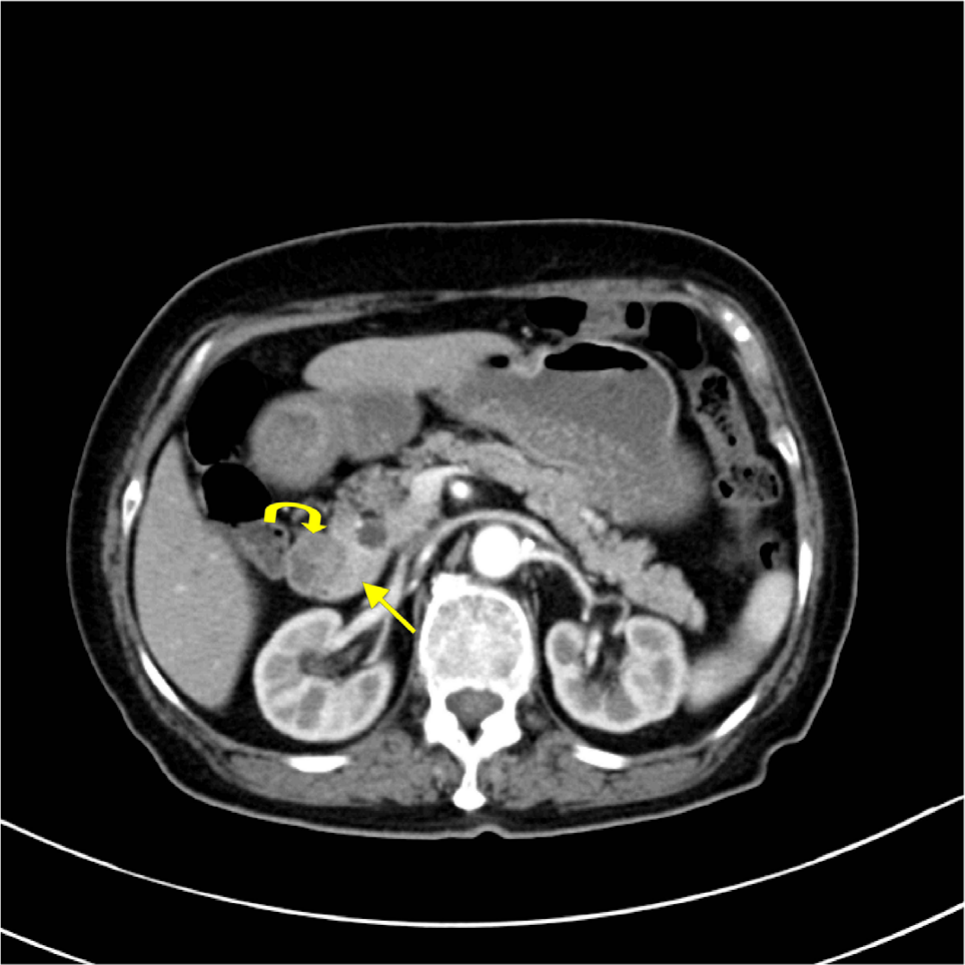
A 71‐year‐old woman with esophageal carcinoma. Axial multiple detector spiral computed tomography (MDCT) arterial phase shows pancreatic tissue (arrow) extending in a posterolateral direction towards the duodenum (curved arrow)
